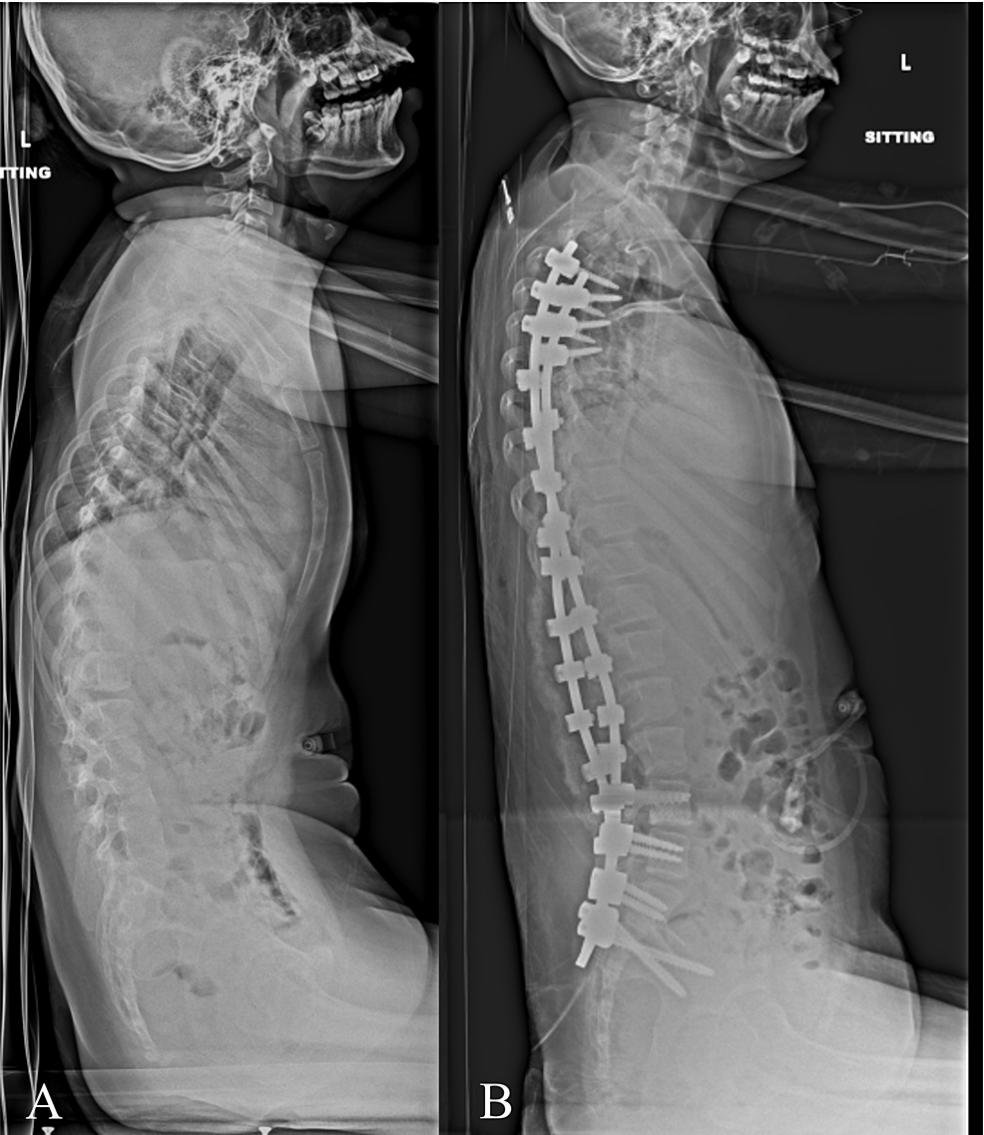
A) Preoperative and B) postoperative sitting lateral x-ray. The preoperative x-ray demonstrates loss of lumbar lordosis (2 degrees), thoracolumbar kyphosis (13 degrees), and positive sagittal balance. The T5-T12 thoracic kyphosis measured 30 degrees. The postoperative x-ray demonstrates T3- pelvis instrumented fusion with the utilization of multiple sublaminal bands with improvement in sagittal balance (T5-T12 thoracic kyphosis 27 degrees, lumbar lordosis 27 degrees, thoracolumbar angle 2 degrees).
